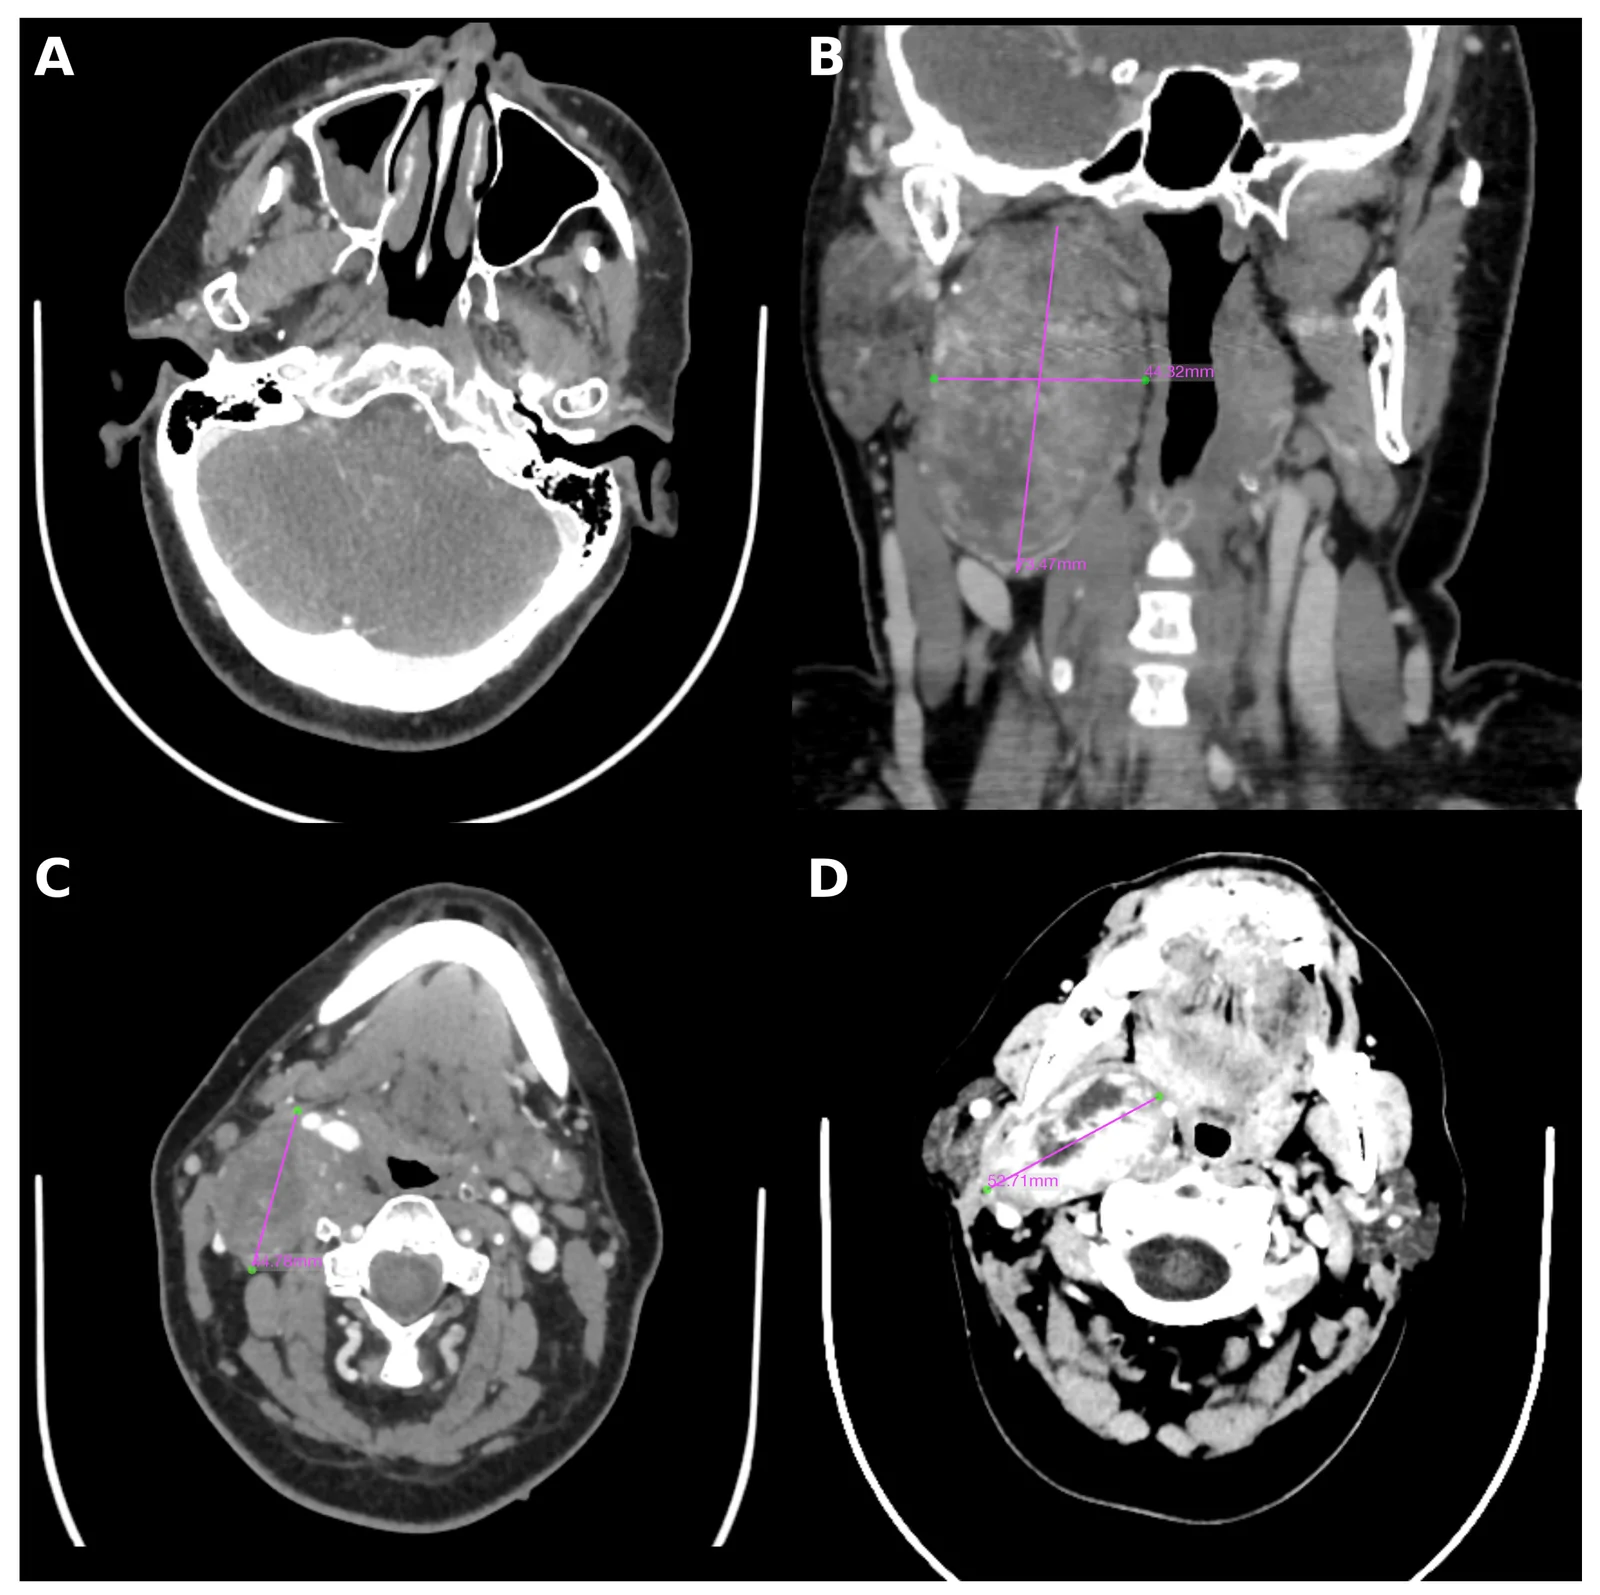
Contrast-enhanced CT scans of the neck were obtained using RadiAnt DICOM Viewer (version 2026.1; Medixant, Poznań, Poland). (A) Axial image at the skull-base level showing the superior component of the right parapharyngeal mass. (B) Coronal arterial-phase reconstruction showing the large craniocaudal extent of the well-defined lesion (approximately 44 mm maximal transverse and 73 mm craniocaudal diameter) and medial displacement of the pharyngeal lumen. (C,D) Axial contrast-enhanced images showing maximal transverse diameter of approximately 53 mm, heterogeneous enhancement and a close relationship with the right carotid–jugular structures. The images were anonymized for publication. The pink lines represent the measurement segments, while the green dots indicate the corresponding measurement endpoints.
